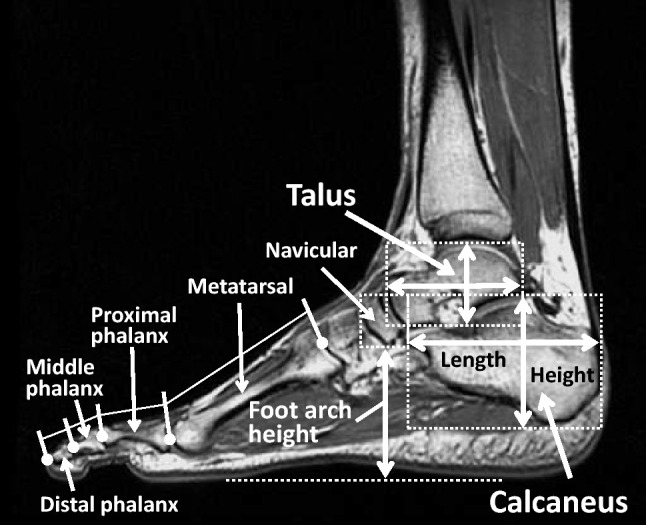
Representative magnetic resonance image for measuring morphological variables of the foot. The lengths of the forefoot bones of the big (included the distal phalanx, proximal phalanx, and metatarsal) and second (included the distal phalanx, middle phalanx, proximal phalanx, and metatarsal) toes were measured along the long axis of the bone between the intersections of the long axis and the cortex at the distal and proximal ends and then totaled to assess overall forefoot bone length for each toe. The lengths and heights of the talus and calcaneus were measured as maximal distances between the cortexes at the distal and proximal ends. The foot arch height was measured as the minimum vertical distance between the navicular and the line connecting the thenar eminence to the bottom of the heel.
